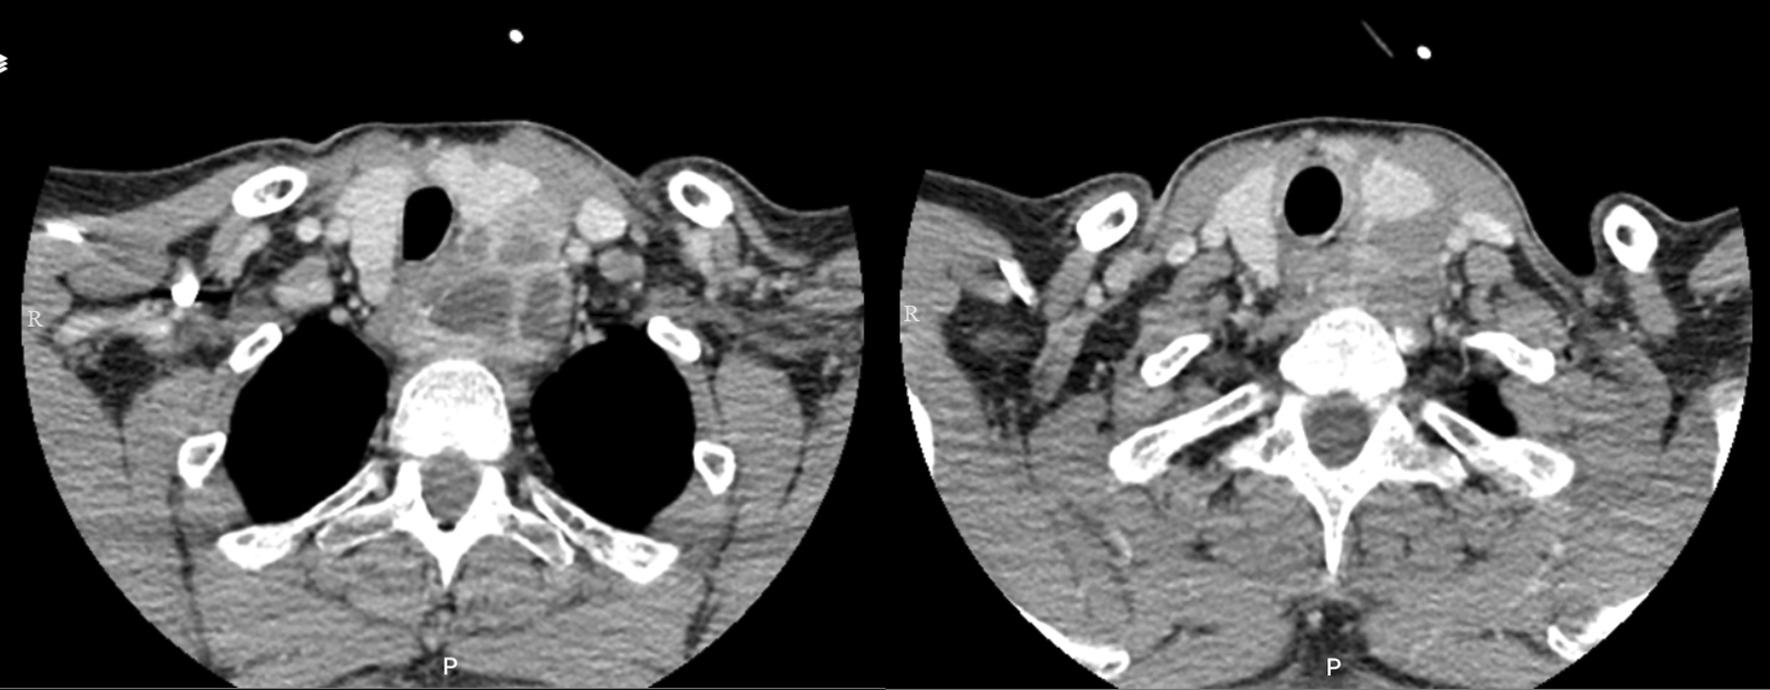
Computed tomography scans of the lower neck (right) and upper mediastinum (left) showing a vast mass pushing the trachea out of the midline
